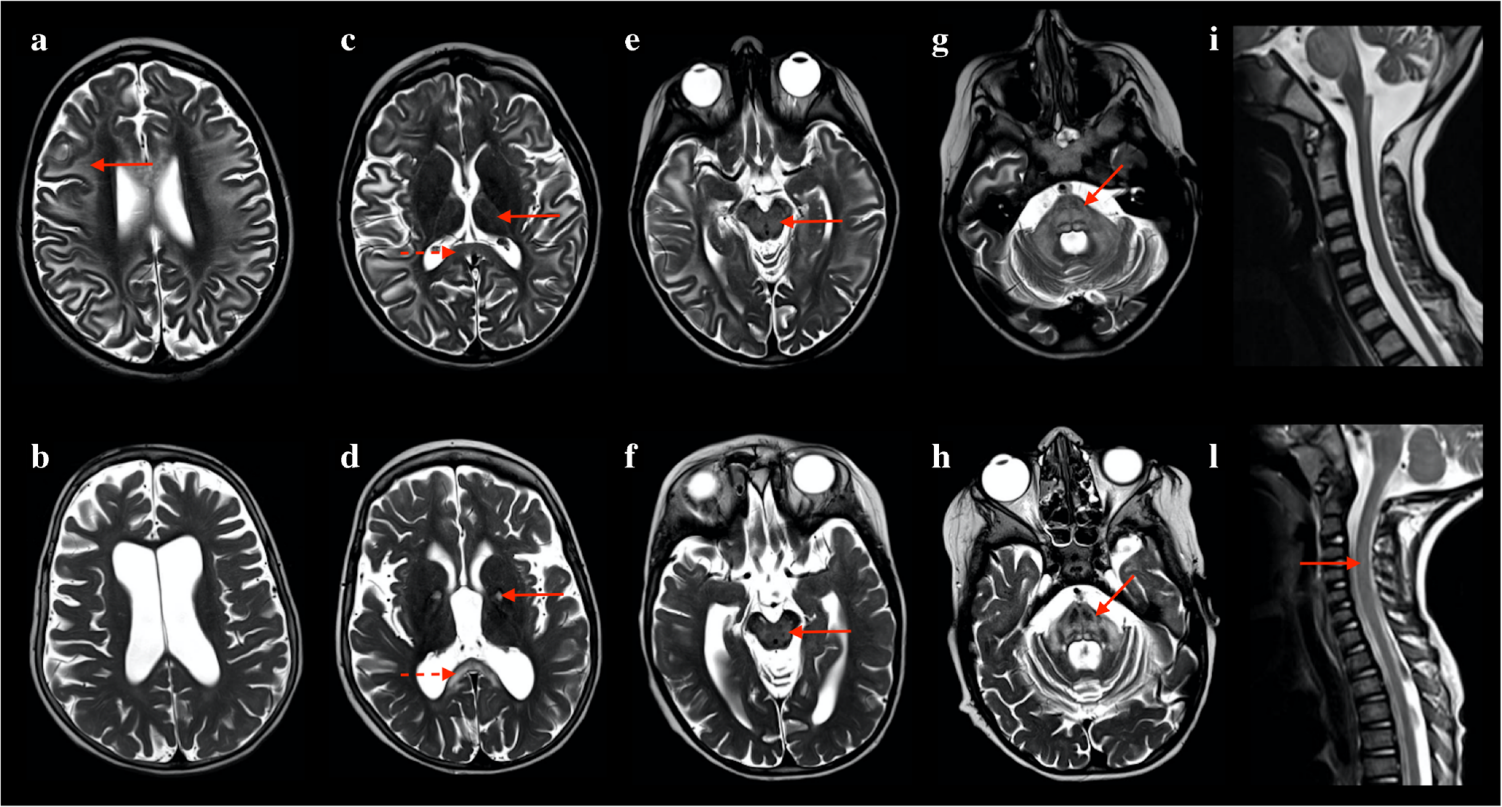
T2-weighted axial and sagittal images of two patients with KSS: the patient above (brain score = 12 points) showed widespread brain involvement, including subcortical white matter (a, solid arrow), thalami (c, solid arrow), and splenium of the corpus callosum (c, dotted arrow), midbrain (e, solid arrow), pons (g, solid arrow), no spinal cord lesions (i). The patient below (brain score = 8) showed slightly less brain involvement, including basal ganglia (d, solid arrow) splenium of the corpus callosum (d, dotted arrow), midbrain (f, solid arrow), pons (h, solid arrow), and spinal cord disease (l, solid arrow)
